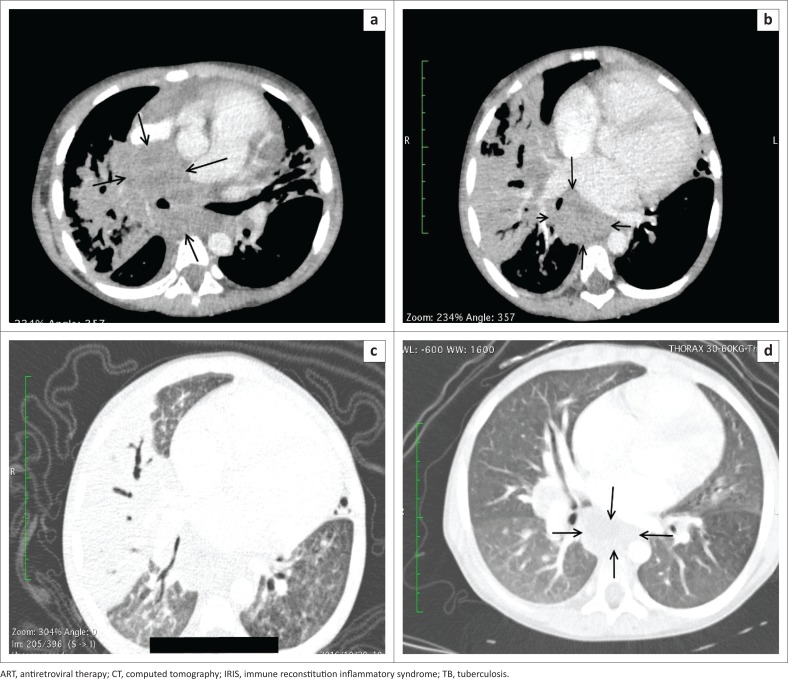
A 4-year-old HIV-infected boy living with an adult diagnosed with pulmonary TB was initiated on ART. Three months later, he developed symptoms of pulmonary TB, which was microbiologically confirmed (Unmasking IRIS). He was initiated on appropriate TB treatment and initially improved; 3 weeks later he presented with fever and worsening respiratory symptoms and signs (Paradoxical IRIS). Computed tomography of the chest, axial, soft tissue window (a and b) demonstrates mediastinal and hilar lymph nodes (arrows) encasing the bronchi bilaterally. There is also posterior mediastinal involvement with anterior displacement of the left atrium and a small right pleural effusion; (c) axial lung window demonstrates dense right middle lobe and apical segment of right lower lobe consolidation with lingular consolidation. A diagnosis of paradoxical and unmasking TB-IRIS was made. ART and TB treatment were continued and corticosteroids were administered; he demonstrated a good clinical response and is currently well; (d) axial CT chest axial lung window performed 6 months later demonstrates resolution of the consolidation, with residual lymphadenopathy (open arrows).
